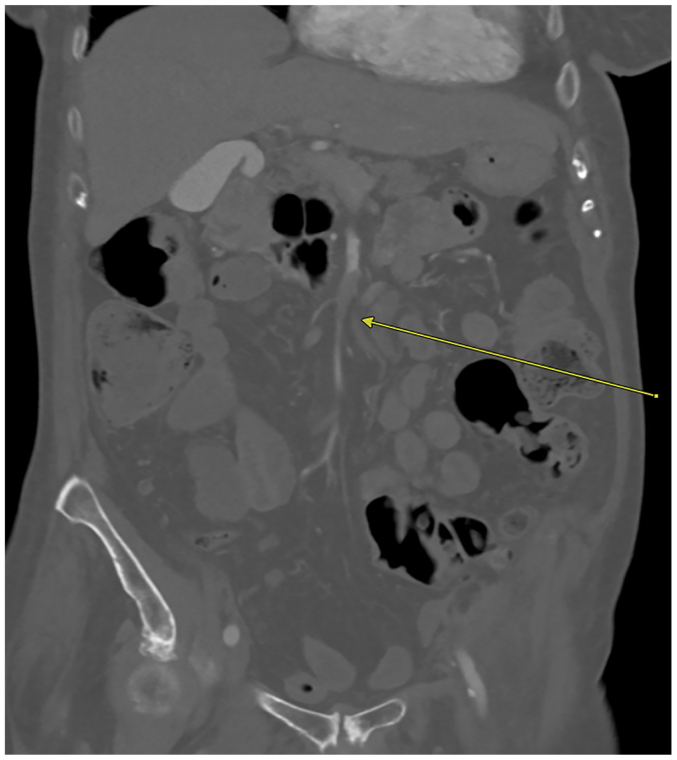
Coronal view of computed tomography angiography (CTA) abdomen/pelvis demonstrating the superior mesenteric artery (SMA) occlusion with distal reconstitution.
